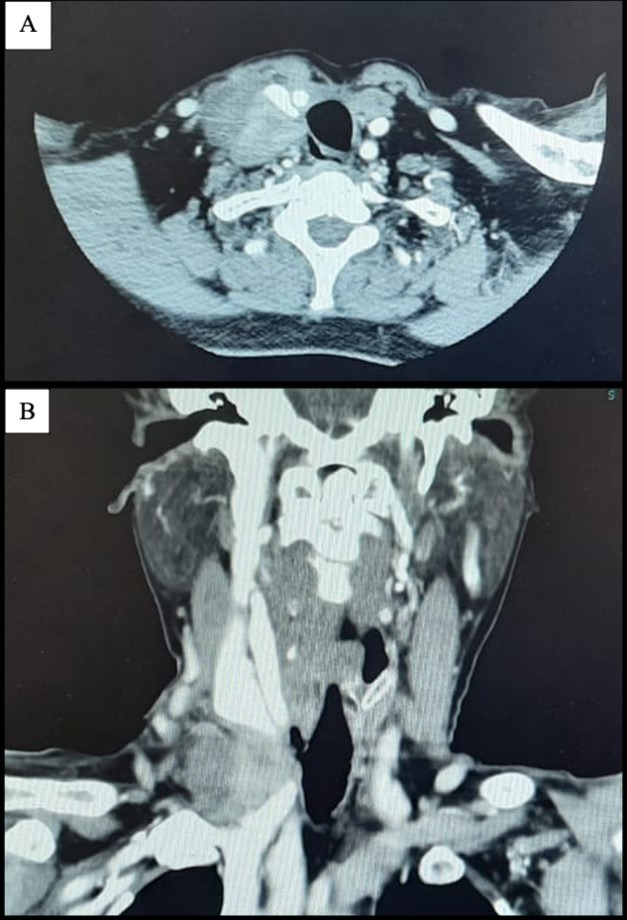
Computed tomography showing: (A) tumor in intimate contact with the posterolateral portion of the right internal jugular vein, esophageal wall, and right sternocleidomastoid muscle (axial slice); and (B) the same tumor and its relationship to the convergence of the right internal jugular vein and the right subclavian vein (coronal slice).
